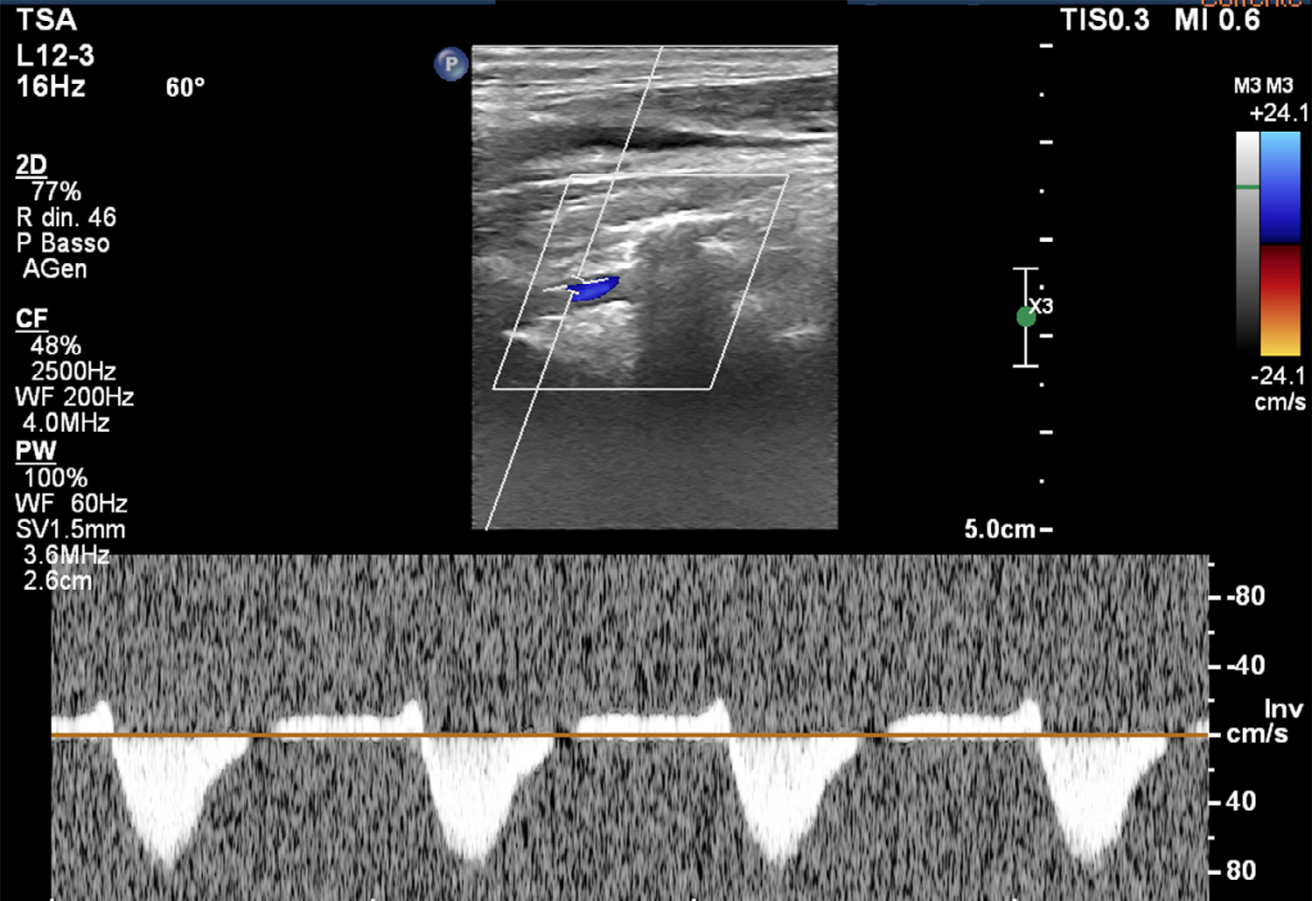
Flow-velocimetric evaluation of the left vertebral artery showing significant flow reversal on echo-color-Doppler examination.
